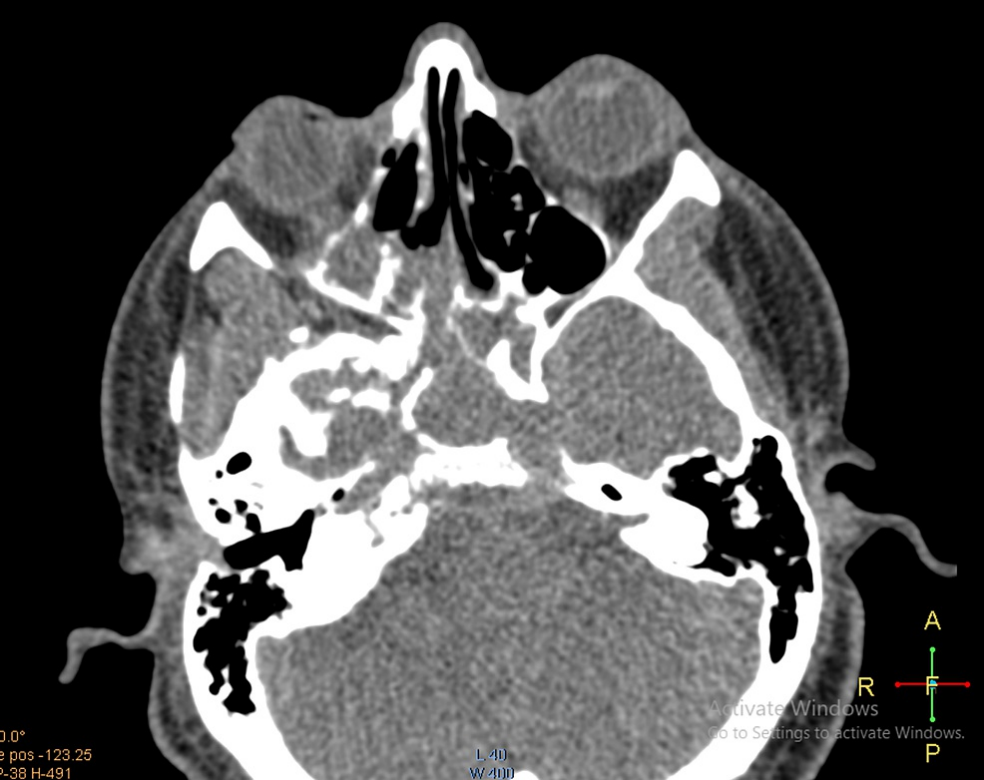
CT of the head axial view showing invasive, left-sided fungal sinusitis. CT: computerized tomography
